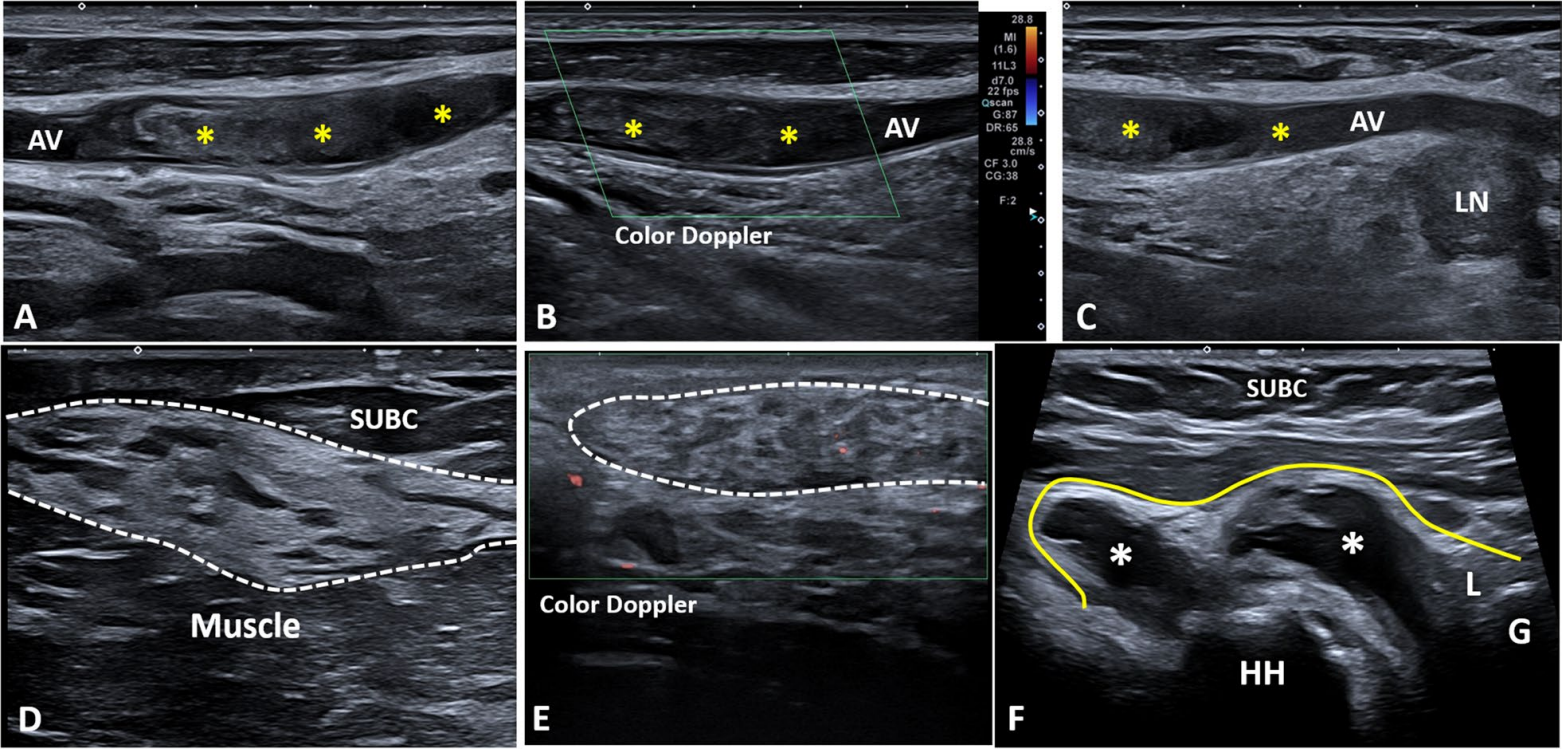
Additional sonographic findings of the axilla. Longitudinal scans (A–C) show a massive thrombosis *(yellow asterisks)* of the axillary vein *(AV)* coupled with a globular lymph node *(LN)* in a patient with neoplastic disease. Accessory breast tissue *(white dotted line)* is usually located in between the subcutis *(SUBC)* and muscle planes (D) showing a peculiar sonographic pattern with hyperechoic fibroductal matrix, hypoechoic fat islands and poor vascular signals in normal conditions (E). Articular effusion *(white asterisks)* distending the axillary recess *(yellow line)* can be easily identified using the standing position in a patient with glenohumeral osteoarthritis (F). *HH* Himeral head, *G* Glena, *L* Labrum
